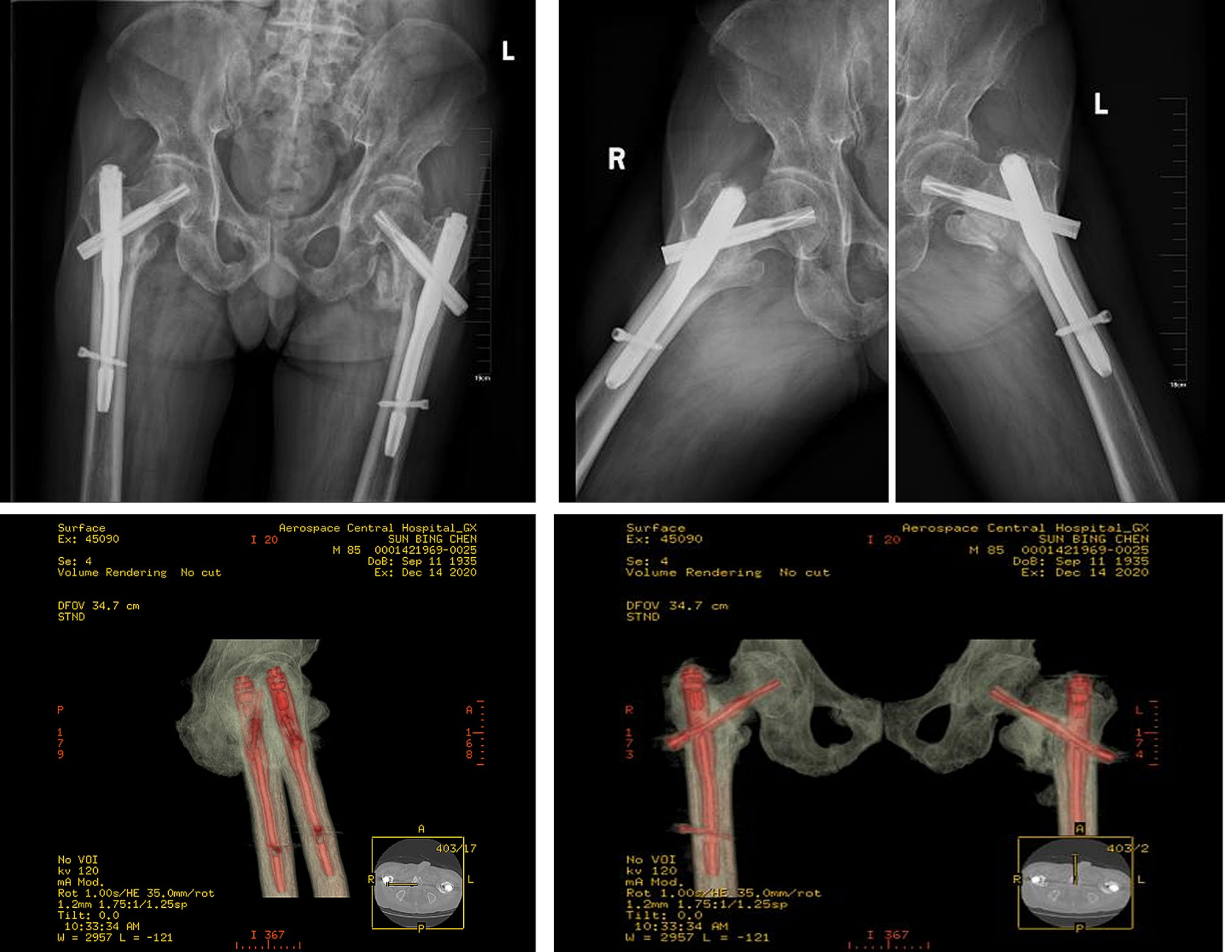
Imaging examination 6 months after surgery. The X-ray and CT showed that the bilateral intertrochanteric fractures of the femur were consistent with healing. The right hip joint was directly healed, with the broken ends of the bones in close contact. There was a large amount of callus formation between the left hip joints, indicating indirect healing
